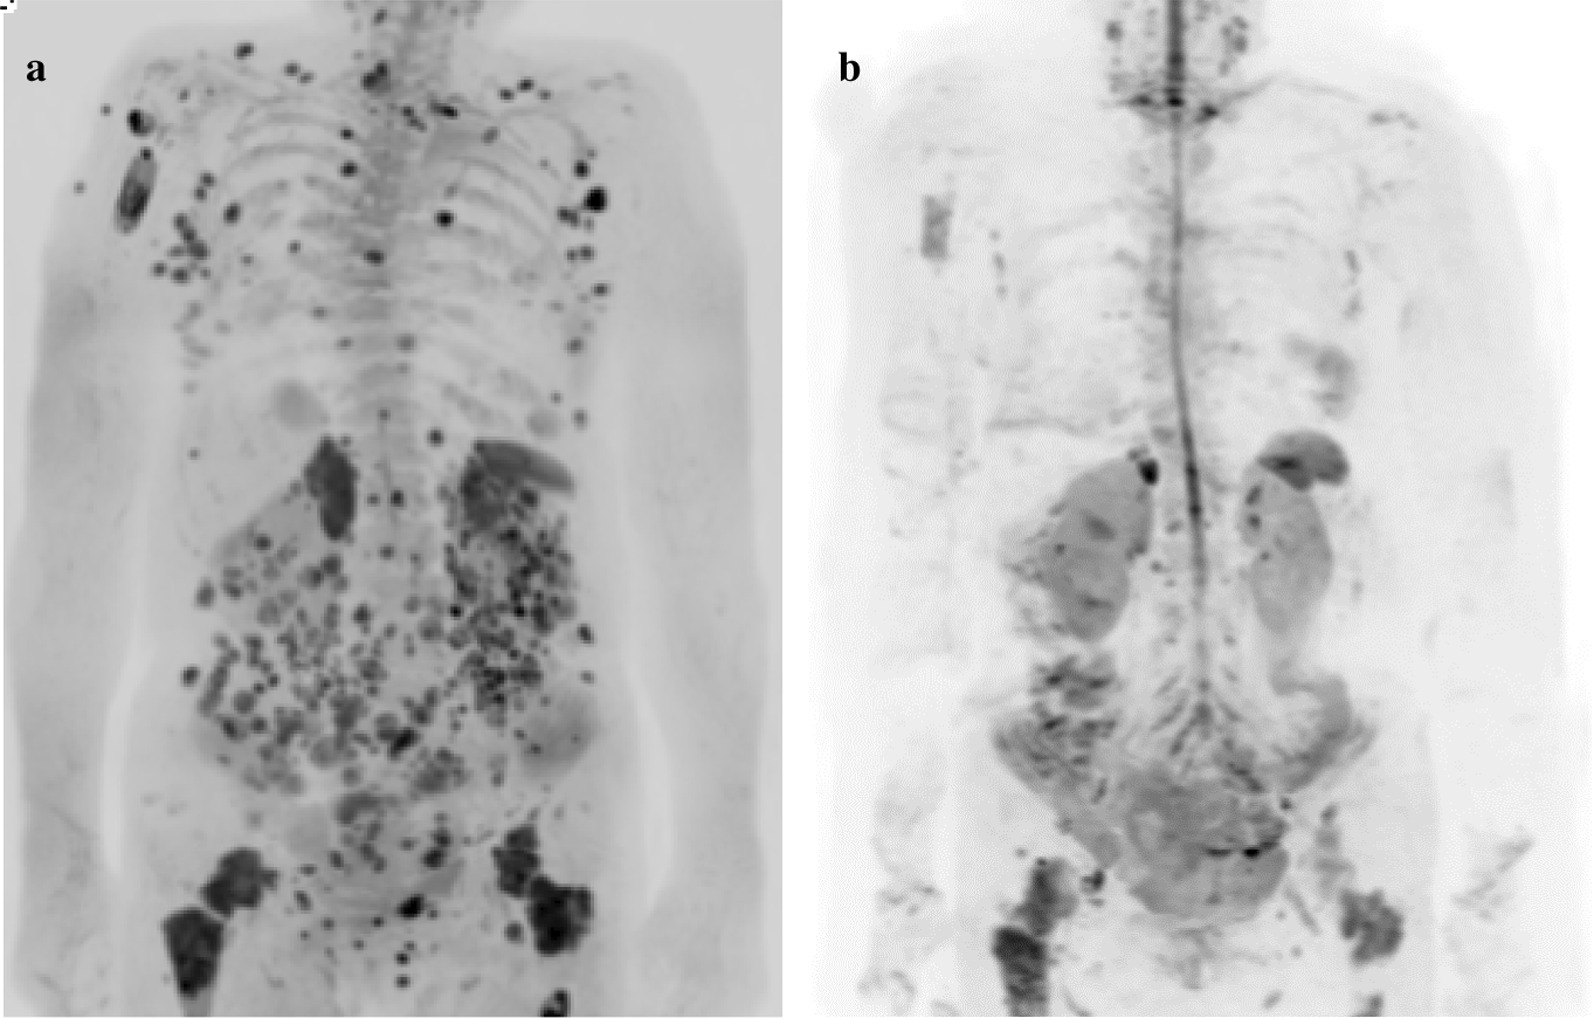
Magnetic resonance imaging (MRI)–diffusion-weighted imaging with background body signal suppression: a multiple lymph node and bone metastases before nivolumab treatment; b good response after eight cycles of nivolumab
